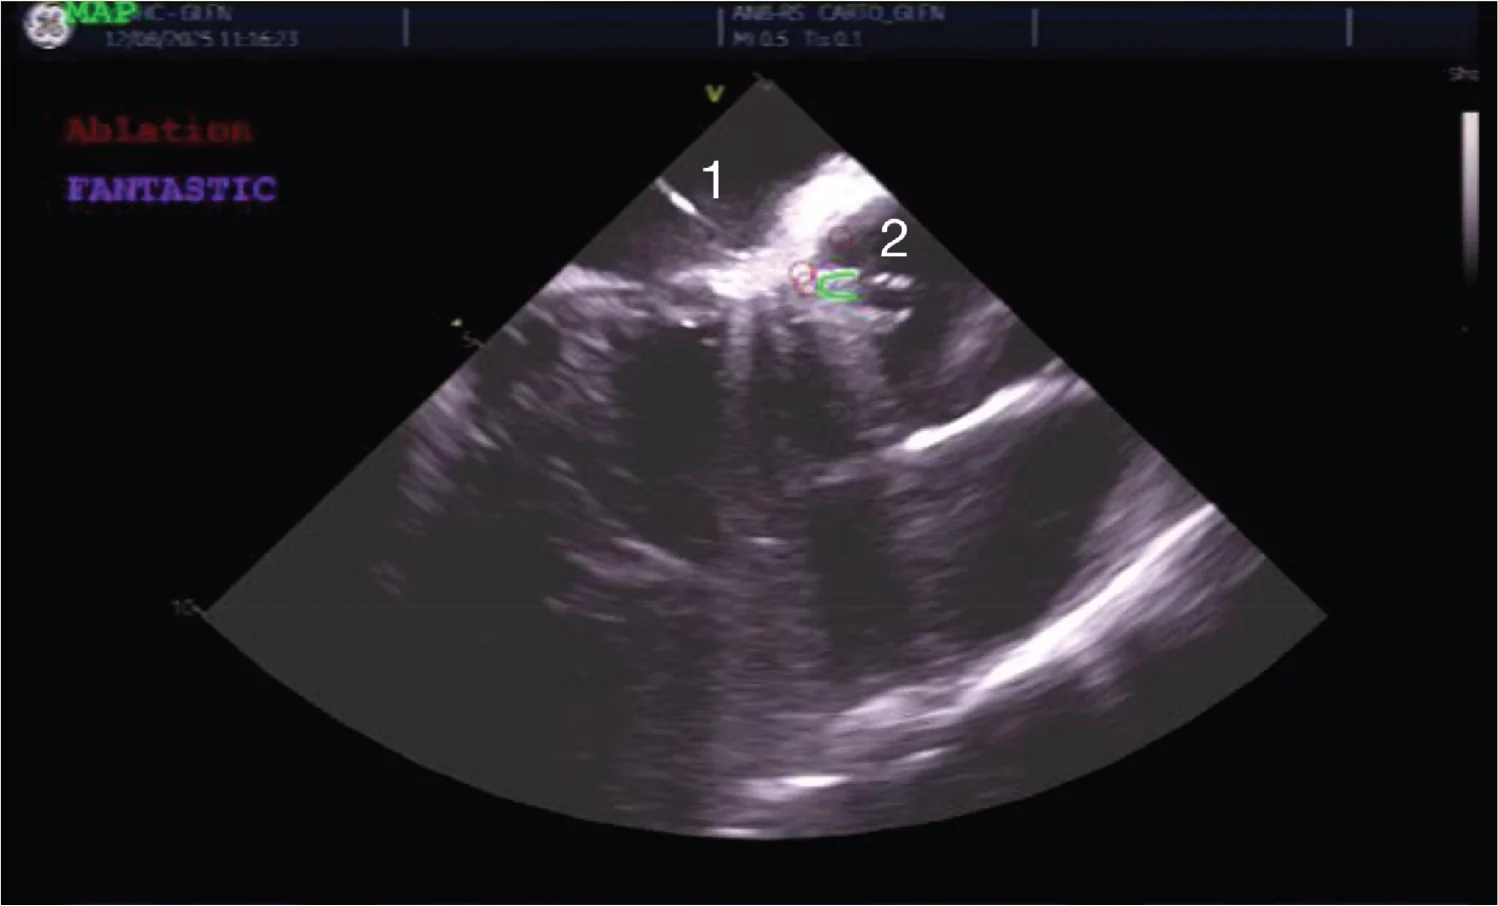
Intracardiac echocardiography showing the quadripolar catheter (1) located in the right atrium at the His region just across the non-coronary cusp (NCC) and the ablation catheter (2) positioned at the NCC of the aortic valve.
